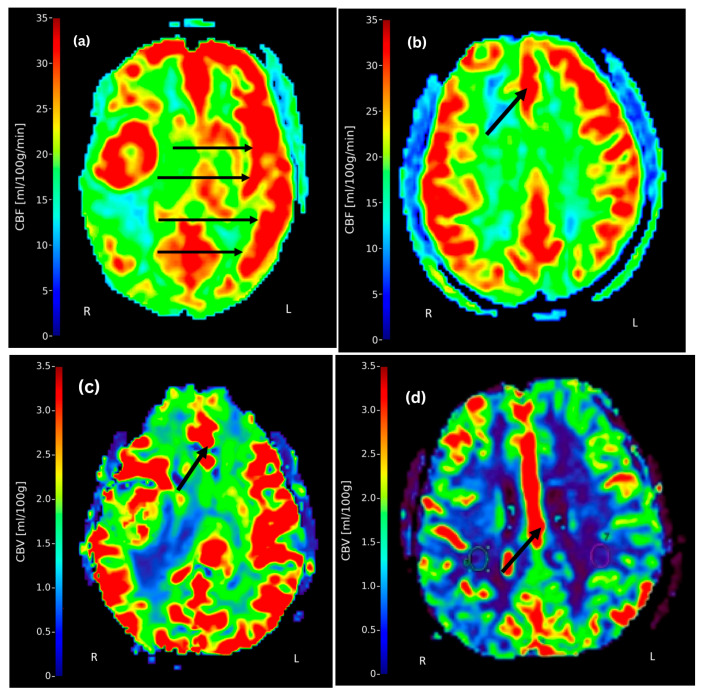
Representative post-contrast DCE-MRI T1-weighted axial slices acquired within 48 h of stroke symptom onset from four different ischemic stroke patients. Panels (a–d) display colour-coded perfusion maps quantifying cerebral blood flow (CBF; range: 0–35 mL/100 g/min) and cerebral blood volume (CBV; range: 0–3.5 mL/100 g). The parameters used for image acquisition and their post-processing are described in Section 2.4. (a) Image from a 63-year-old patient showing left-sided dominance of CBF in the middle cerebral artery (MCA) territory, as indicated by arrows. (b) Image from a 51-year-old patient showing elevated CBF in the left anterior cerebral artery (ACA) territory (arrow). (c,d) images from 26- and 75-year-old patients, respectively, showing high CBV in the left ACA territory (arrow). These visual findings are consistent with the results of the paired *t*-test analyses, which demonstrated significant hemispheric asymmetries in CBF and the descriptive analysis of CBF and CBV. The arrows highlight perfusion dominance or reduction regions, visually confirming the hemispheric asymmetries and perfusion variations quantified through descriptive (Table 3 and Table 4) and inferential (Table 5) statistical analyses.
